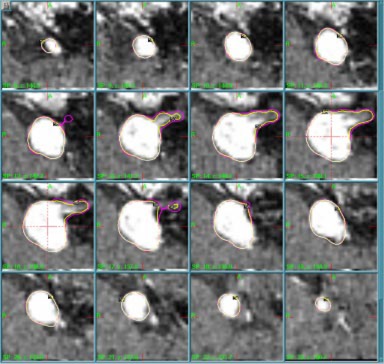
(Color) Treatment plan for an acoustic neuroma patient. Displayed are 16 sequential 1‐mm‐axial MR images through the enhancing target. Displayed are the resulting 50% isodose levels obtained if ${\text{OF}}_{4/18}$ is the same (purple) or 20% smaller (yellow) than that input into the treatment planning system (${\text{OF}}_{4/18}=0.870$).
